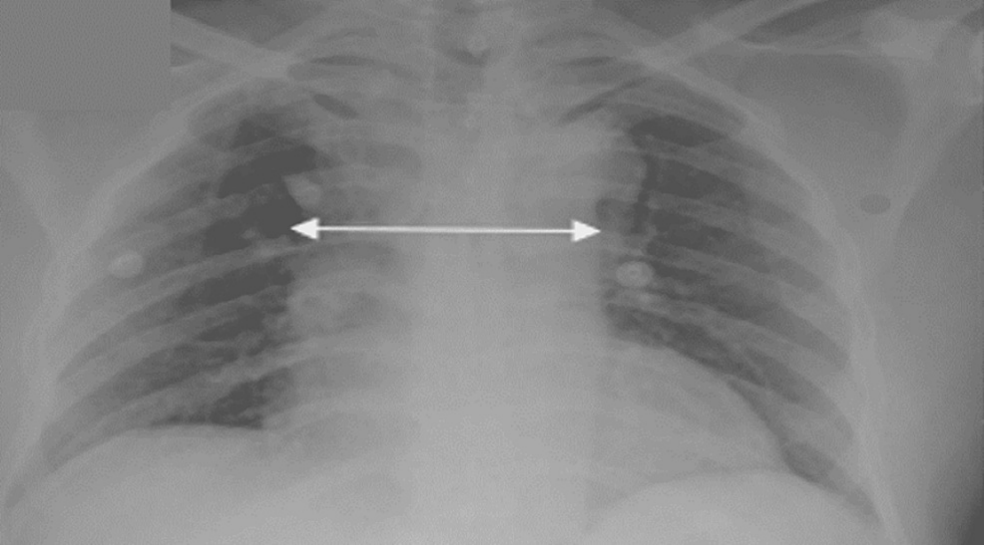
Chest X-ray in the semi-sitting position showing the widening mediastinum (arrow).
